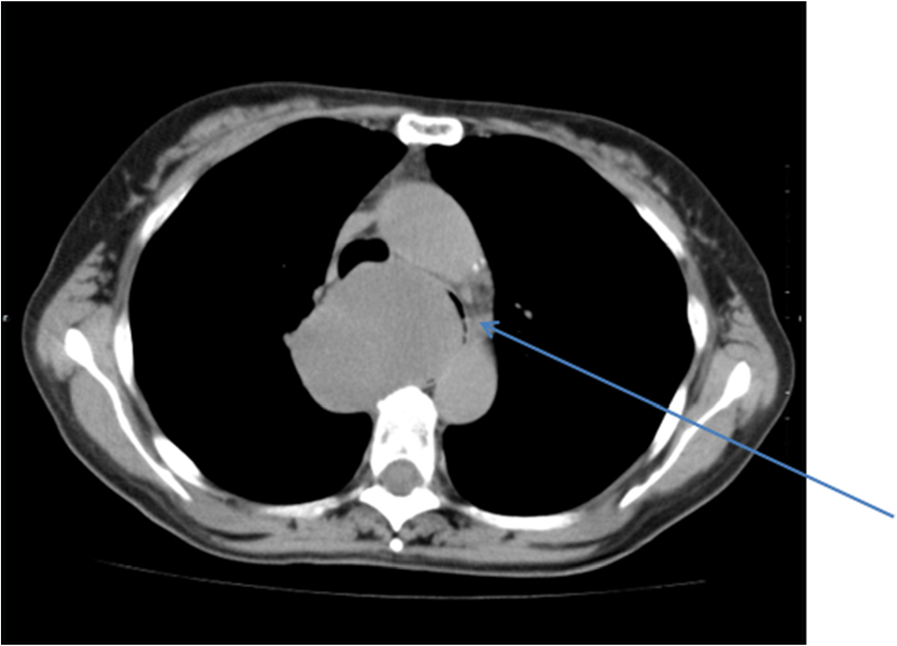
Chest computed tomography (CT) scan showed a lobulated tumor (75 × 57 × 80 mm) in the upper to middle mediastinum. The arrow shows compression of the esophagus.
